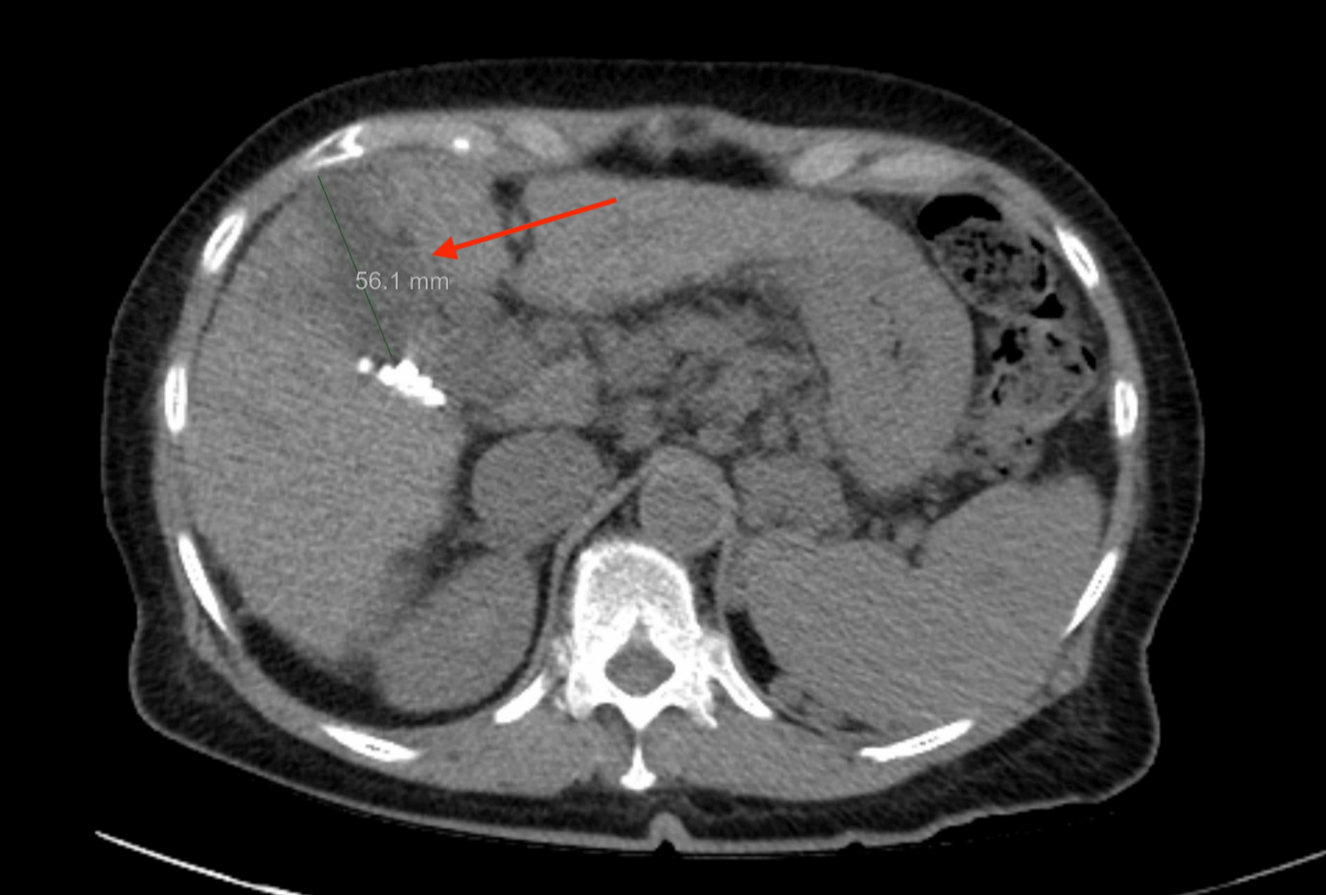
Axial CT of the abdomen and pelvis with contrast revealing suspected HCC (arrow) CT: computed tomography, HCC: hepatocellular carcinoma
